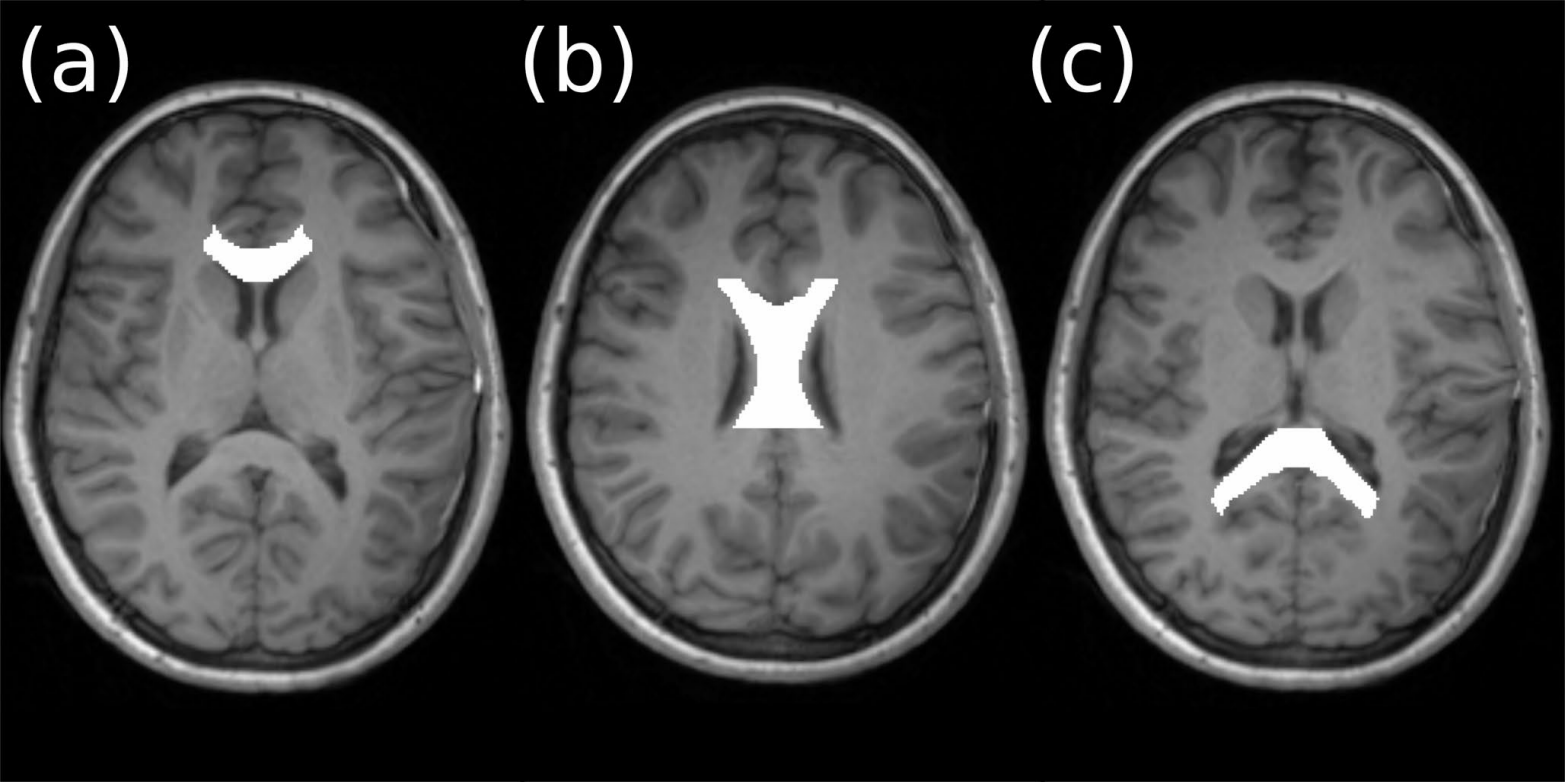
3D T1-weighted images displayed in the axial plane. The regions of interest displayed on the images represent the parcellations of the corpus callosum used in the analysis. (a) genu, (b) body and (c) splenium
